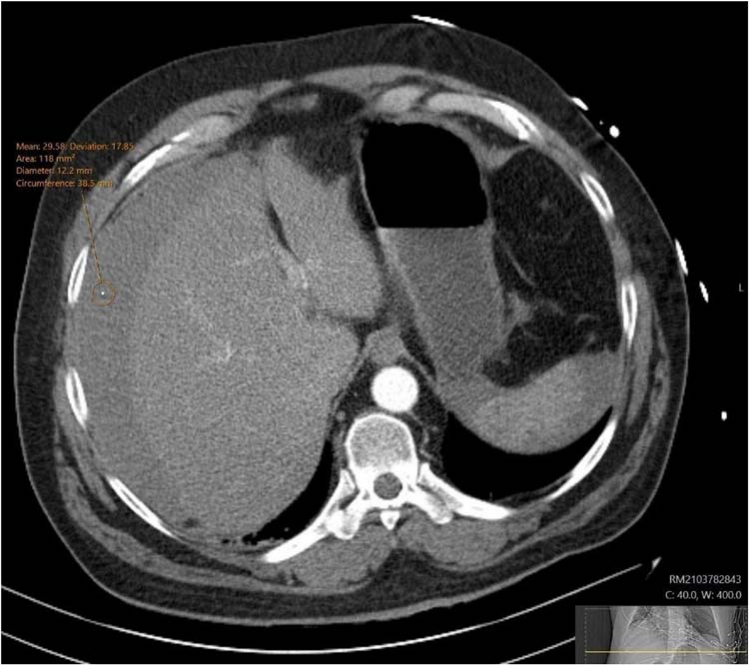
CT angiogram showing a peri-hepatic haematoma. Figure 2 is an axial section taken from the patient’s initial CT angiogram of the abdomen and pelvis. A large crescent shaped peri-hepatic haematoma is highlighted by the arrow displayed.
